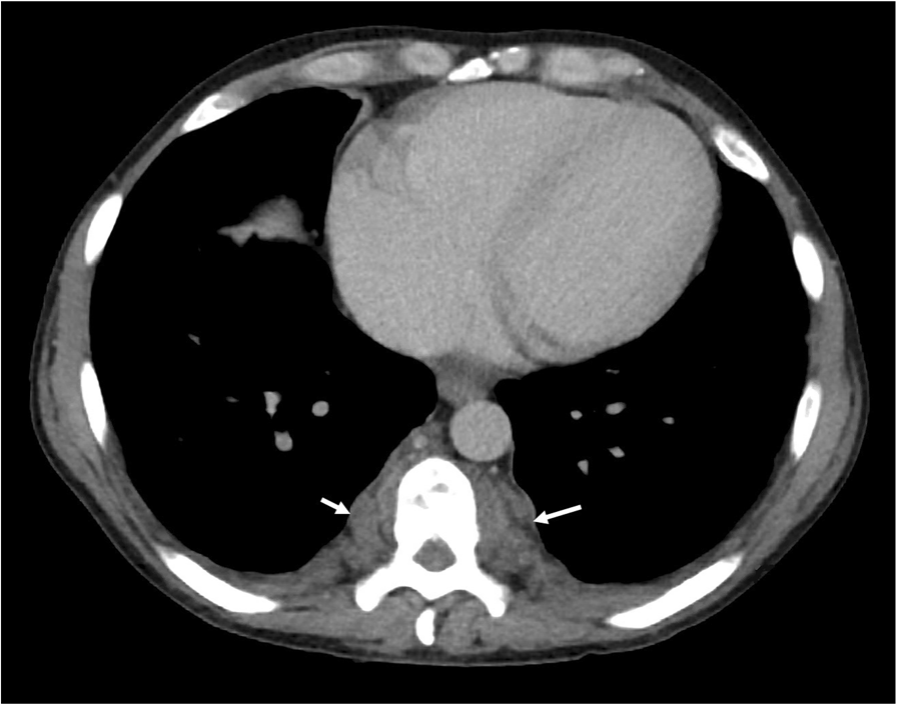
Axial CT of the chest demonstrating extramedullary haematopoiesis in the posterior mediastinum, which typically appear as bilateral and symmetrical posterior mediastinal masses (white arrows). In the paravertebral regions, the soft tissue may compress the exiting nerves in the neural exit foramina or enter the vertebral canal, causing cord displacement or compression
